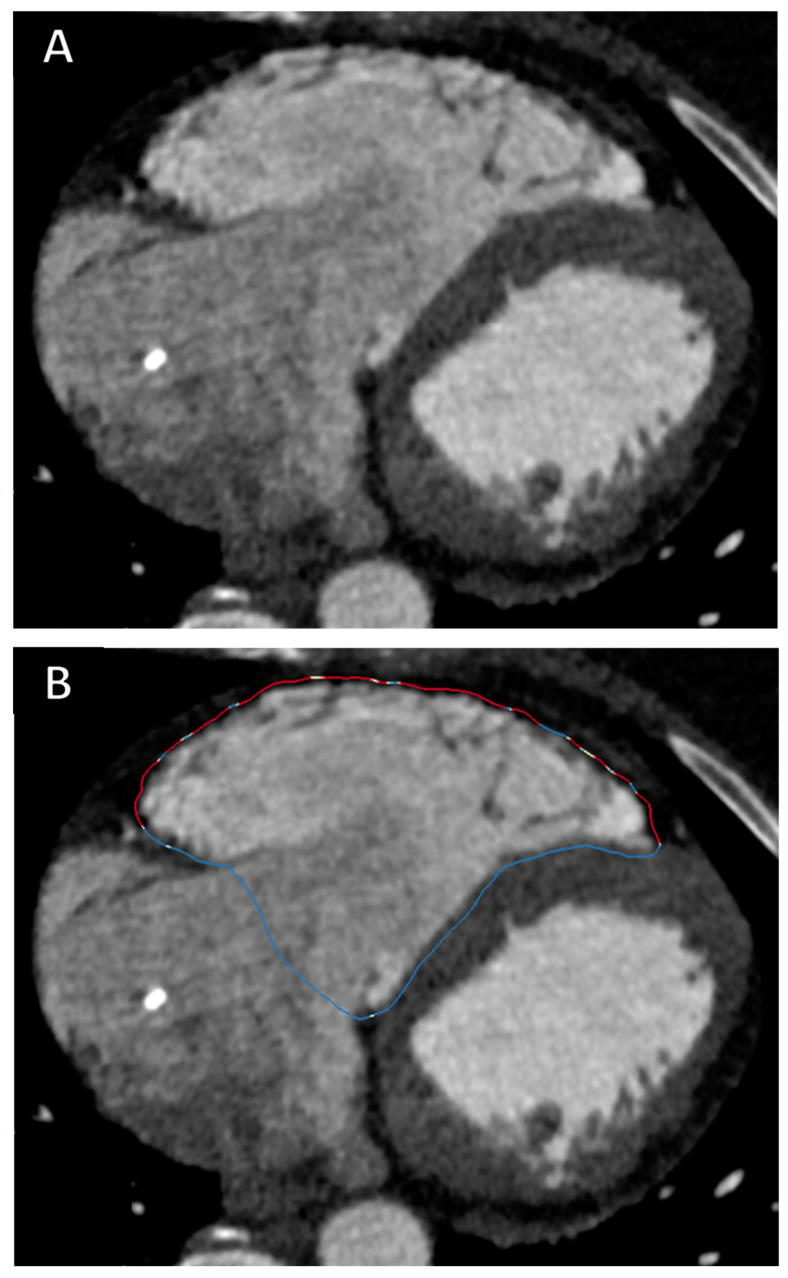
Panel (A) shows CT image of a patient with ARVC; the RV is dilated plus wall thinning and hypoattenuations of the RV lateral wall are clearly visible. Panel (B) shows the RV border drawn by ADAS-3D used to calculate scar parameters. The colours of the border indicate the type of myocardial tissue: blue is healthy myocardium, red is dense scar, white-orange is border zone.
